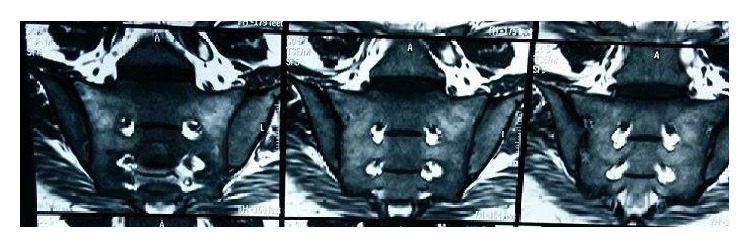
MRI of the sacroiliac joints with bilateral bone marrow edema.
